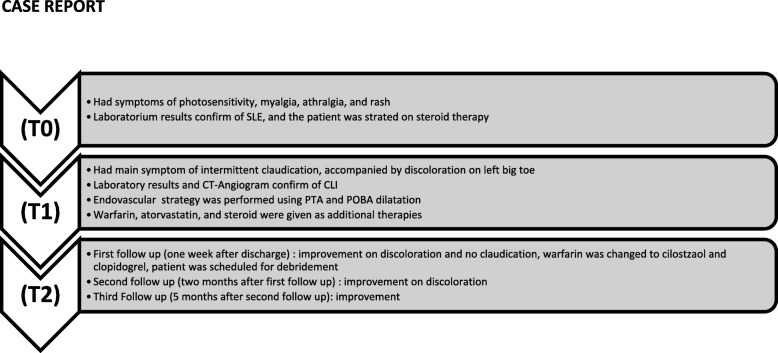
Timeline of the disease. *CLI* critical limb ischemia, *CT* computed tomography, *POBA* plain old balloon angioplasty, *PTA* percutaneous transluminal angioplasty, *SLE* systemic lupus erythematosus, *T0* 1 month prior to admission, *T1* on admission, *T2* on follow-up
